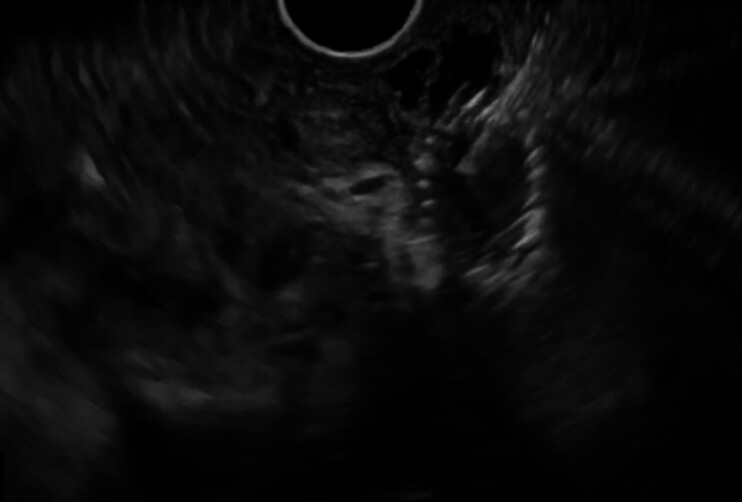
The stent placed for endoscopic ultrasound-guided hepaticogastrostomy was punctured using a 19-G needle.
